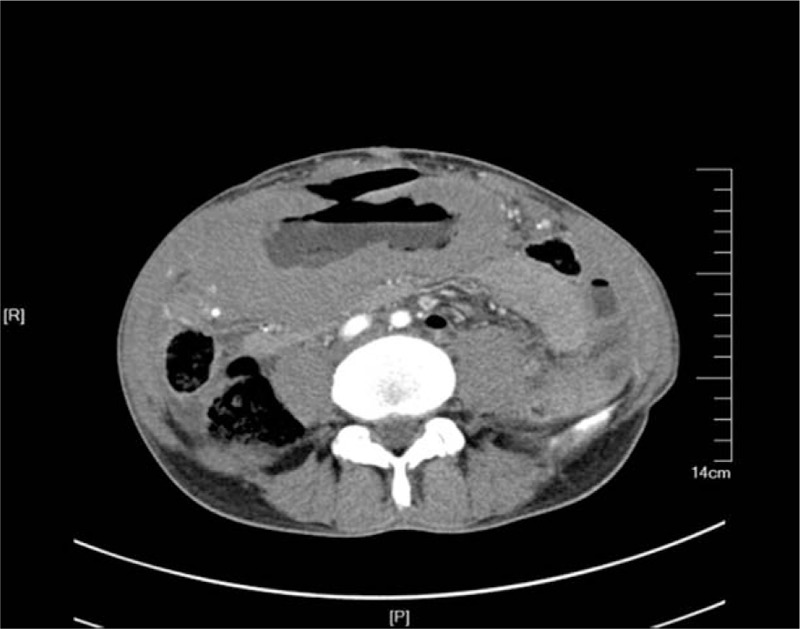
Abdominal CT showed that a crack can be seen on the antetheca of gastric antrum and the gastric wall was diffusely incrassated.
